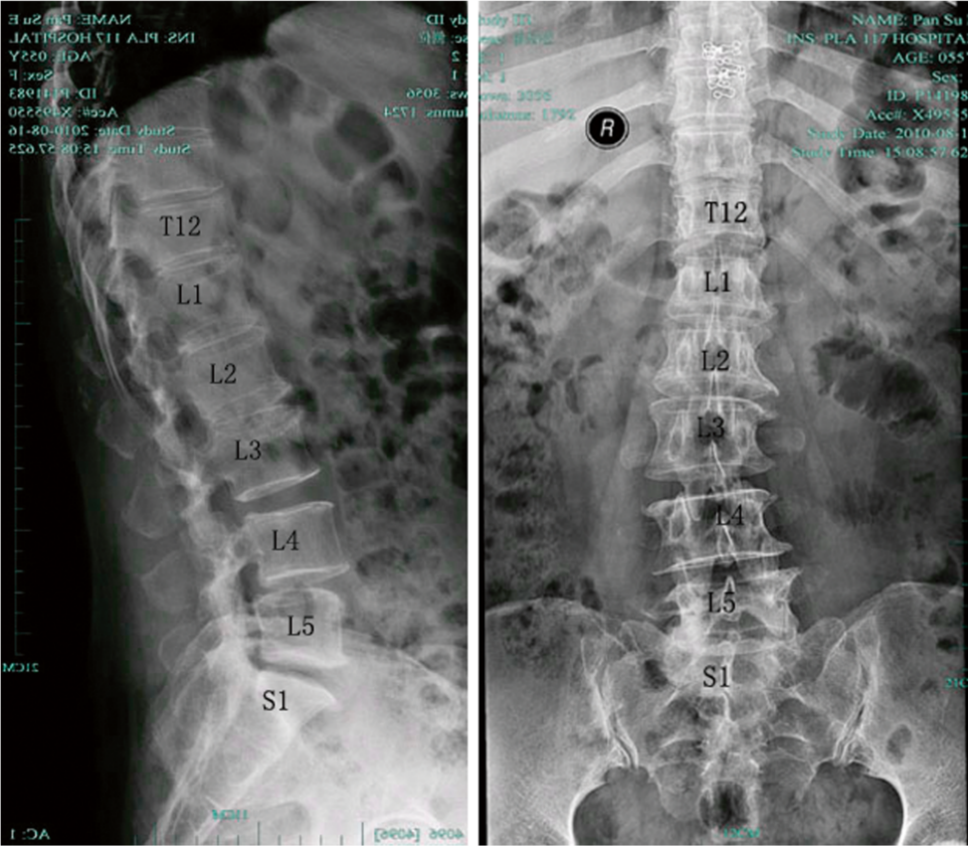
DS patient’s X-ray plate of L-Spine PA and LAT with coronal Cobb angle about 10° and L4 spondylolisthesis and vertebra osteophyte
